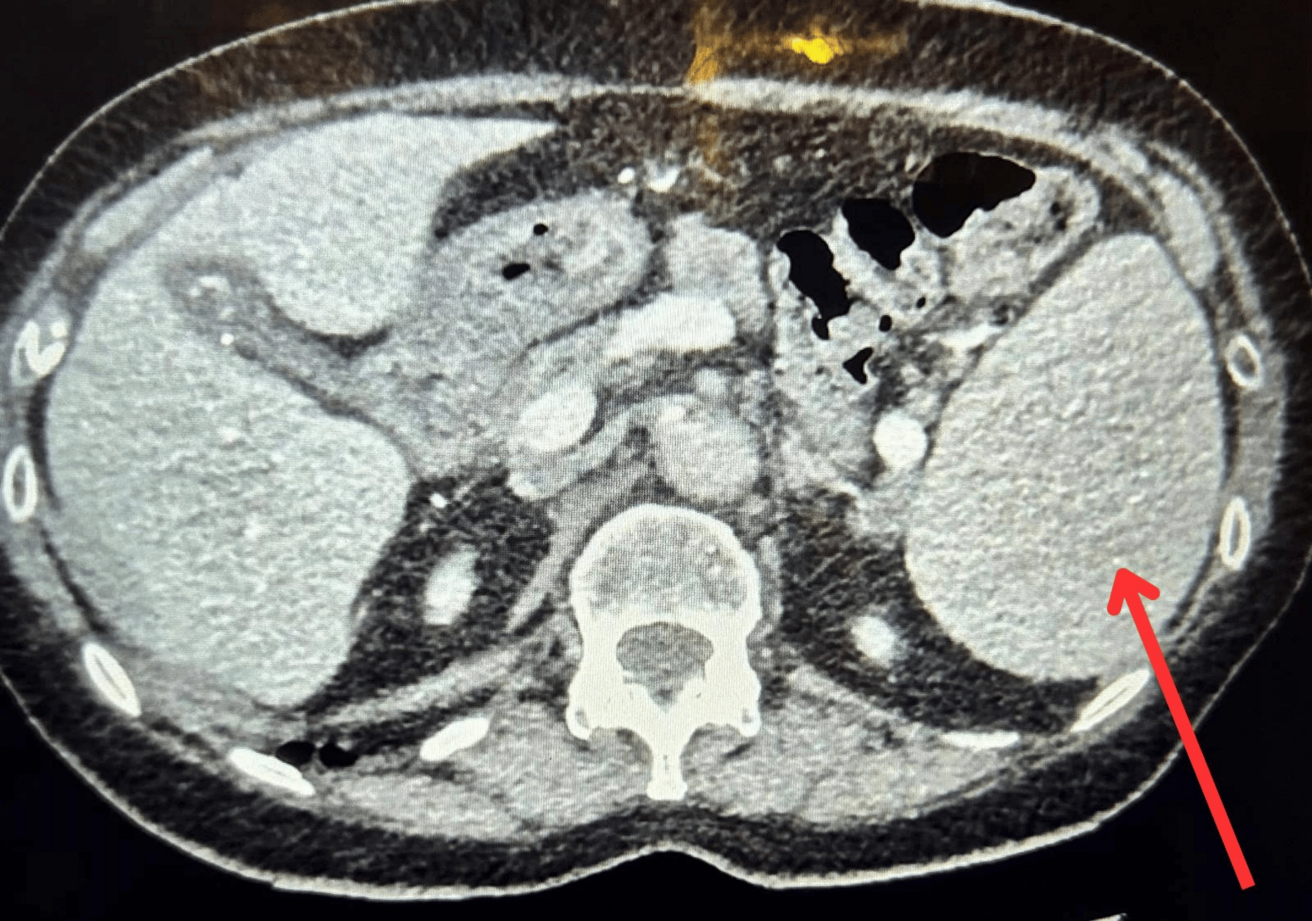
CT scan of the thorax, abdomen, and pelvis shows splenomegaly
